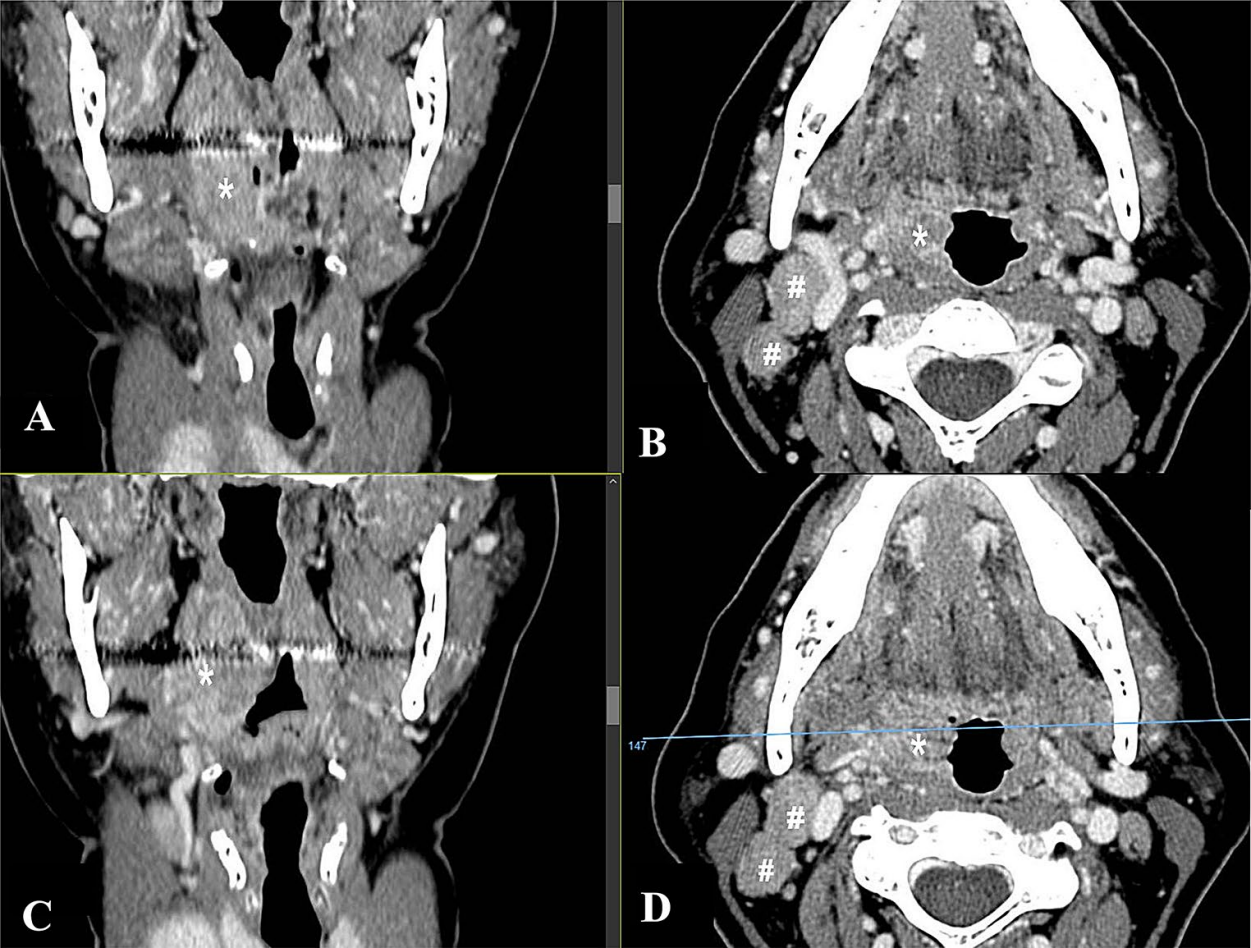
A-B-C-D Radiological images (case 1). (A-C) CT images with contrast enhancement coronal views and (B-D) axial views. The star (*) indicates the right palatine tonsil, where pathological infiltration of the right tonsillar fossa is evident. The infiltration extends downward along the palatoglossal fold and tapers off toward the ipsilateral glossoepiglottic vallecula. The hash symbol (#) highlights multiple pathological lymph nodes at levels II and III on the right side, with the largest node presenting an axial diameter of 2 cm
